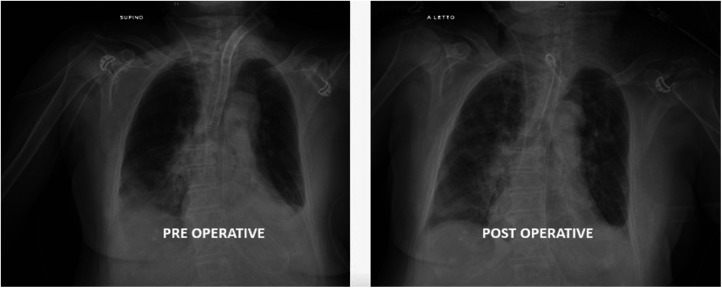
An adequate tracheal caliber and the presence of the new tracheostomic cannula can be appreciated at the post-operative x-ray. The mechanical ventilation system showed no air losses in the circuit at the end of surgery.
